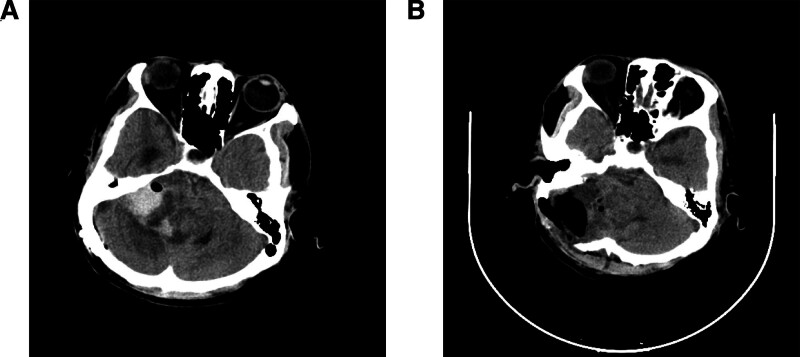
(A) CT showed cerebellar swelling and hematoma on March 10. (B) Postoperative imaging showed the hematoma was cleared. CT = computed tomography.
